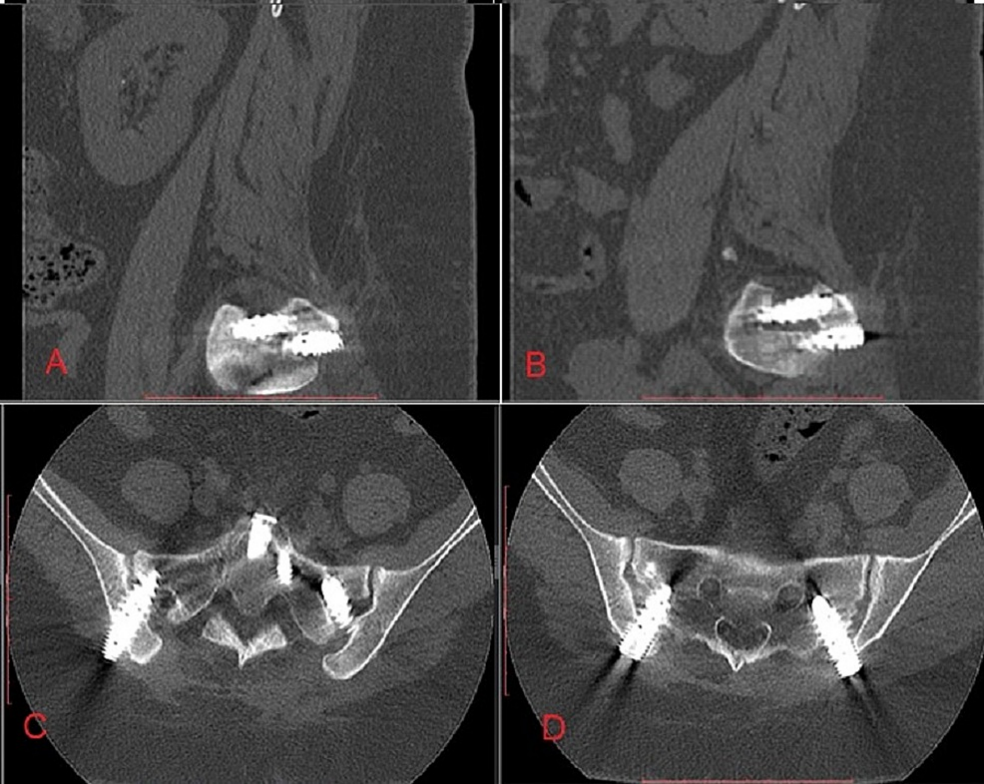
Postoperative CT scan showing fusion A: Sagittal slice left; B: Sagittal slice right; C: Axial slice S1 vertebrae; D: Axial slice S2 vertebrae
